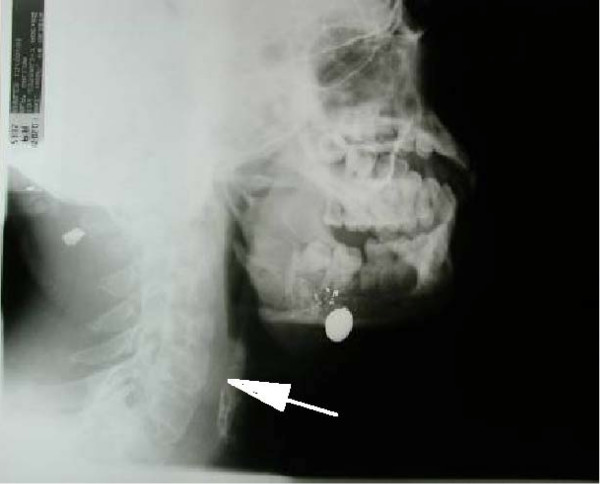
Male patient who sustained low velocity missile injury to the left chick. Lateral radiography demonstrates the bullet location. Note the patent airway on the lateral view (white arrow).
